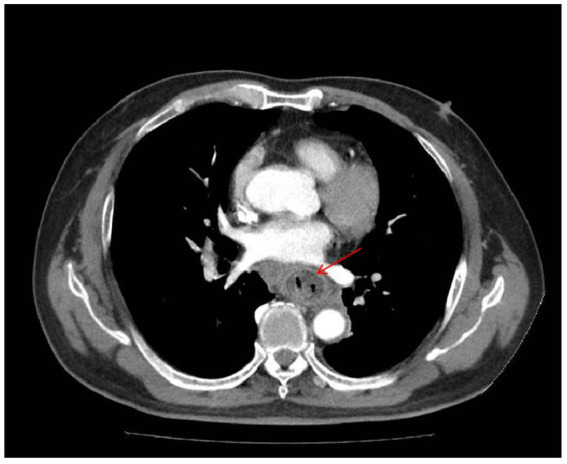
CT showed diffuse thickening with oedema of the oesophageal wall (red arrow indicates the lesion).
